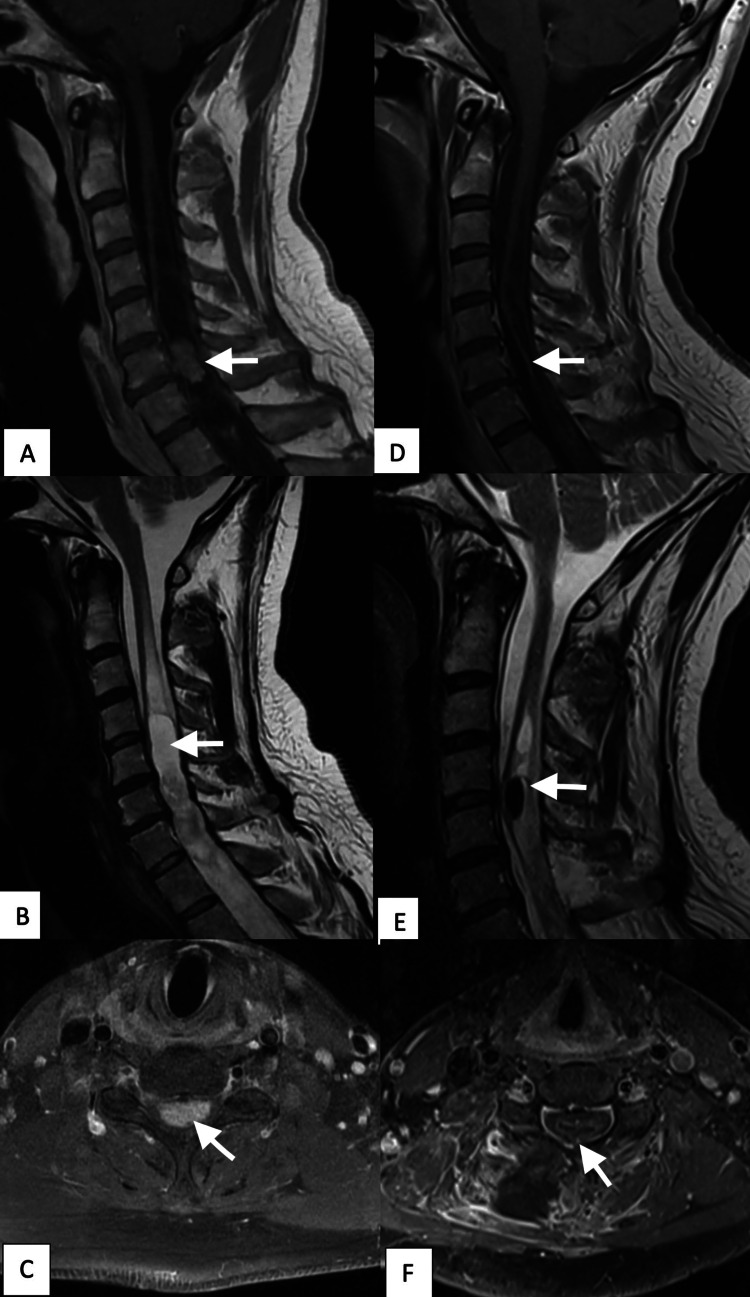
Preoperative and postoperative cervical MRI. (A) Preoperative T1-weighted MRI with Gadolinium injection showing enhancing intramedullary tumor at C6 level (arrow). (B) Preoperative T2-weighted sagittal MRI showing the syrinx (arrow). (C) Preoperative T1-weighted axial MRI with Gadolinium injection showing the tumor (arrow). (D) Post-operative T1-weighted sagittal MRI with Gadolinium injection showing complete resection of the tumor (arrow). (E) Post-operative T2-weighted sagittal MRI showing the regression of syrinx (arrow). (F) Post-operative T1-weighted axial MRI with Gadolinium injection showing complete resection of the tumor (arrow).
